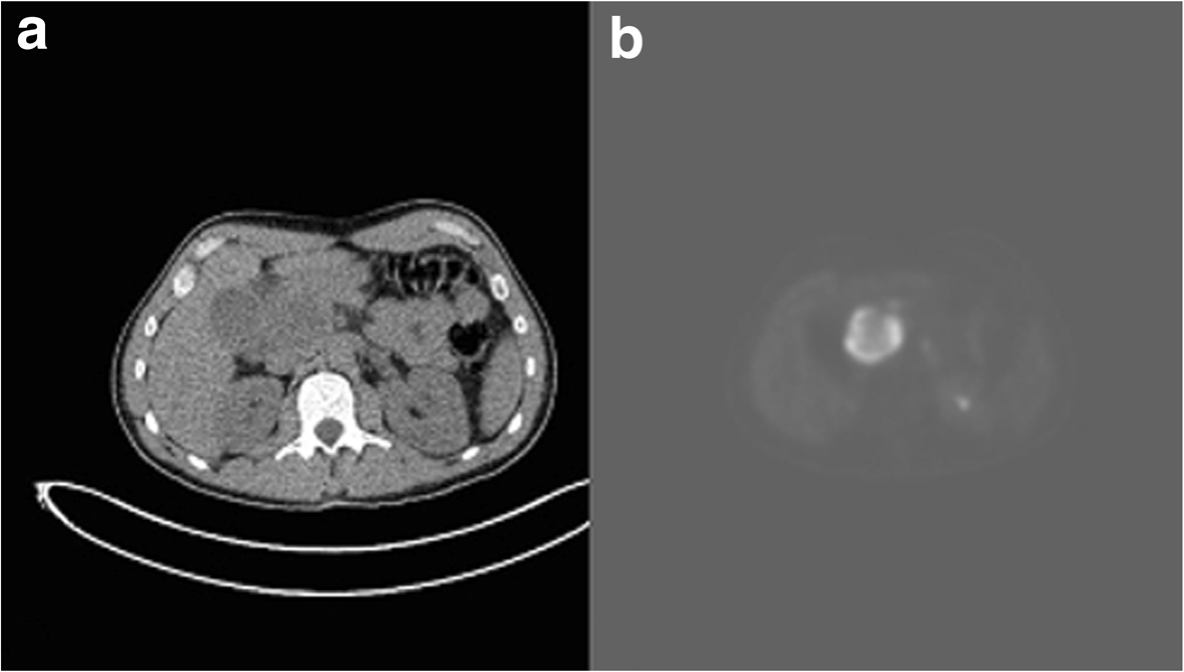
a Abdominal CT showing a large periportal nodal mass and b Abdominal PET-CT showing avid tracer uptake
